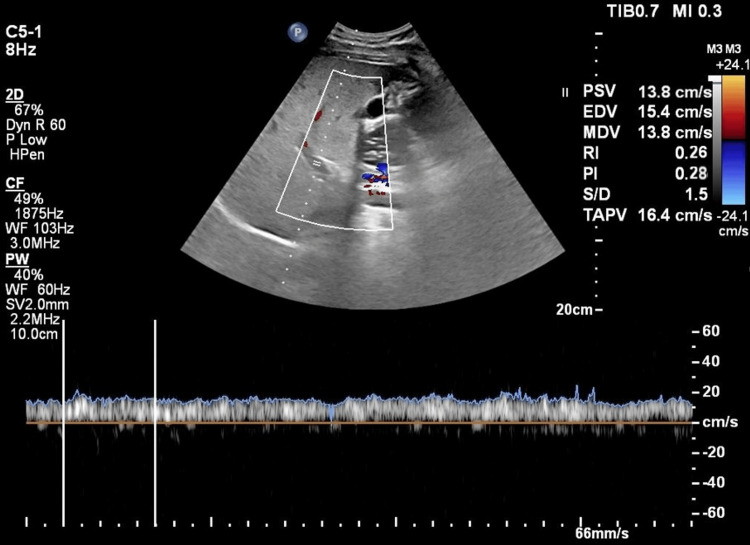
Ultrasound shows Grade 1 fatty liver changes with pulsatility index (PI): 0.28, resistivity index (RI): 0.26, and peak systolic velocity (PSV): 13.8 cm/s, indicating mild hepatic steatosis.
